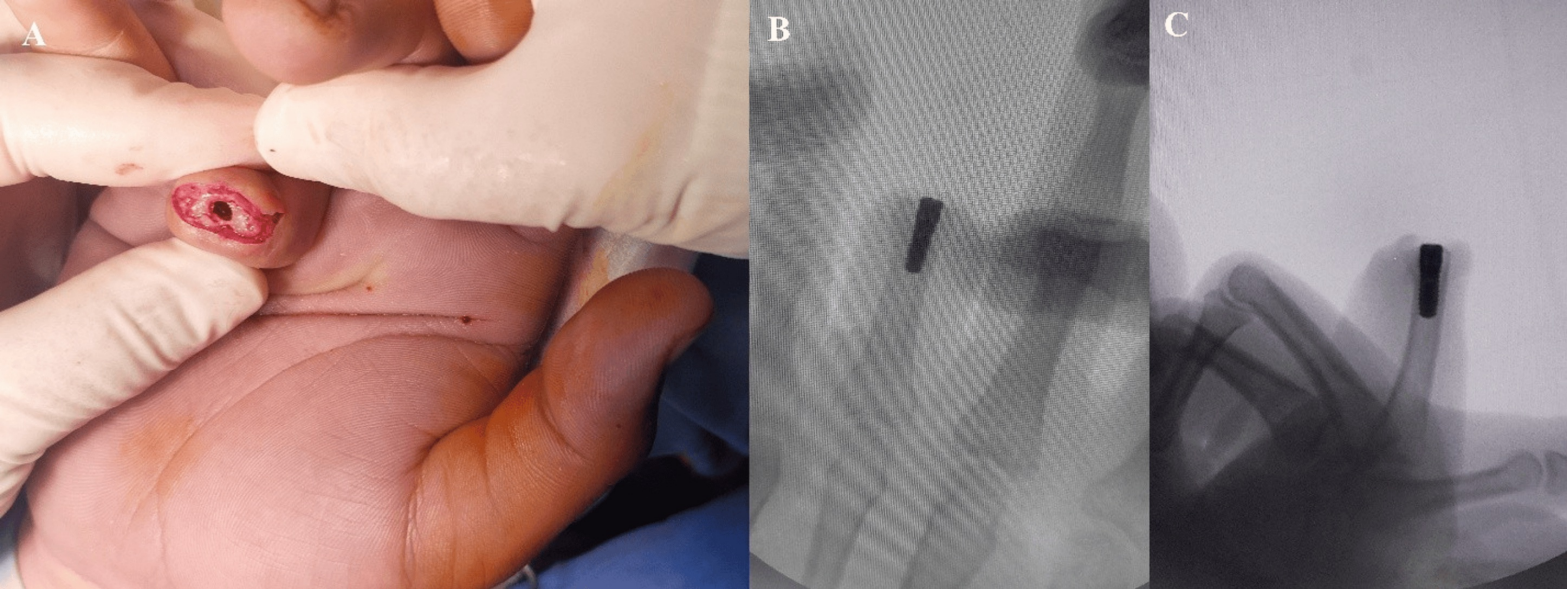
(A) Intraoperative image showing preparation of the medullary canal of the proximal phalanx for dental implant insertion. (B, C) Radiographic images demonstrating appropriate positioning of the dental implant.
